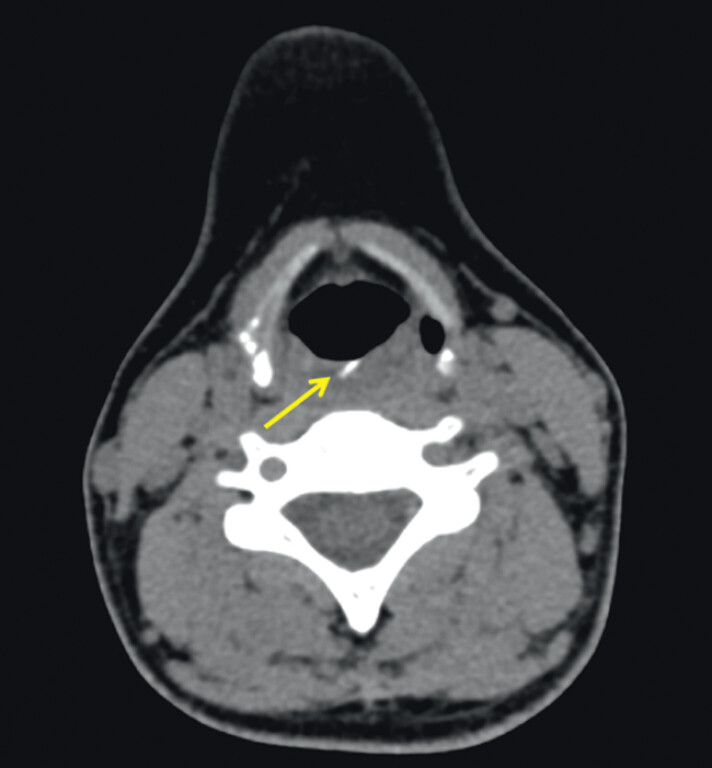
Computed tomography showed a high-density shadow (yellow arrow) about 2 cm in length located at the level of the esophageal entranceway.
